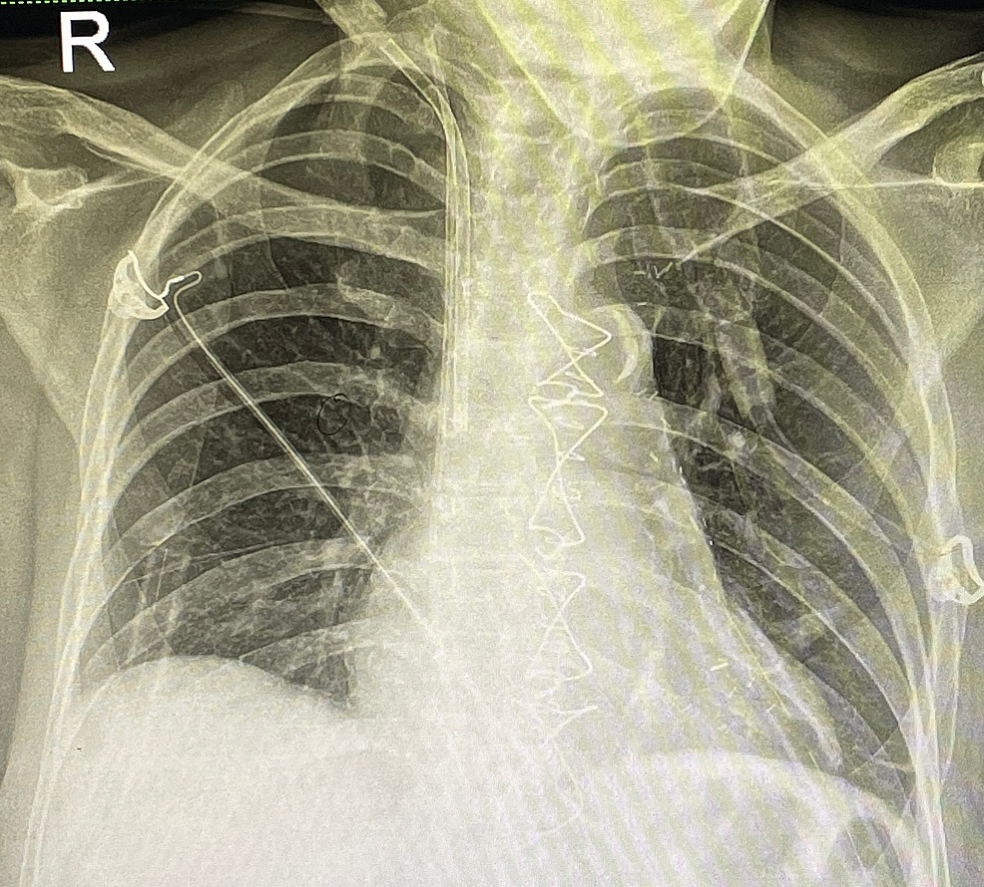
Postoperative chest X-ray showing no recurrent lesion.
